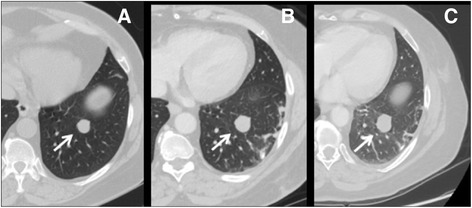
Serial chest CT images showing a representative left lower lobe metastasis over time (arrows). The nodule progressed for 3 months prior to ipi/nivo (image (a) to image (b)) but remained relatively stable 7 months following immunotherapy (image (c)). Three additional pulmonary metastases followed similar clinical courses with no new disease
